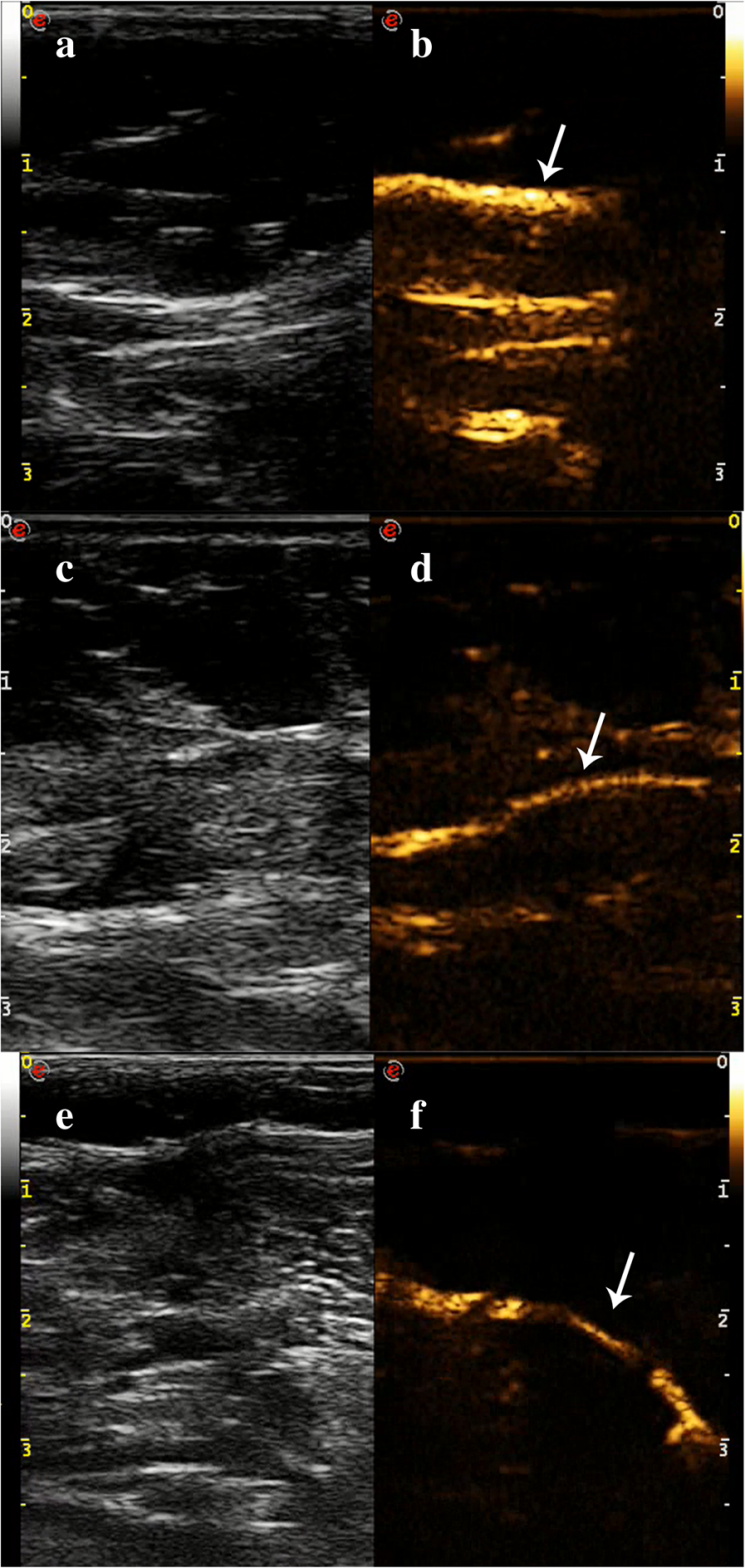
Three kinds of SLCs shown on live dual images. Live dual images can provide images of gray-scale ultrasound and CEUS simultaneously, which could help to identify the enhanced SLCs. Three kinds of SLCs were observed on live dual images in real time: a SSLC which could not be observed on a gray-scale imaging (a) but was enhanced on a contrast-specific imaging (b, *arrow*); a PSLC which could not be observed on a gray-scale imaging (c) but was enhanced on a contrast-specific imaging (d, *arrow*); a DSLC which could not be observed on a gray-scale imaging (e) but was enhanced on a contrast-specific imaging (f, *arrow*)
